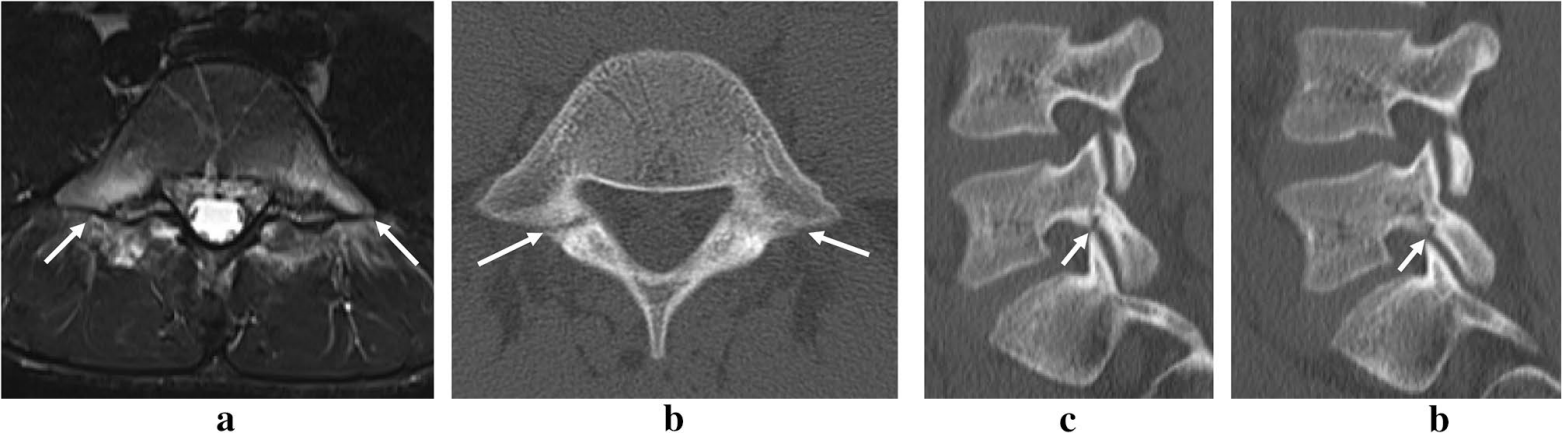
The short tau inversion recovery magnetic resonance images (STIR-MRI) at the first examination showed high intensity change bilaterally (white arrows) in the pedicles of L5 (a). CT revealed bilateral faint fracture lines (white arrows) on the axial slice (b) and slight bone resorption (white arrows) on the ventral side of the lamina in the sagittal view (c, d).
